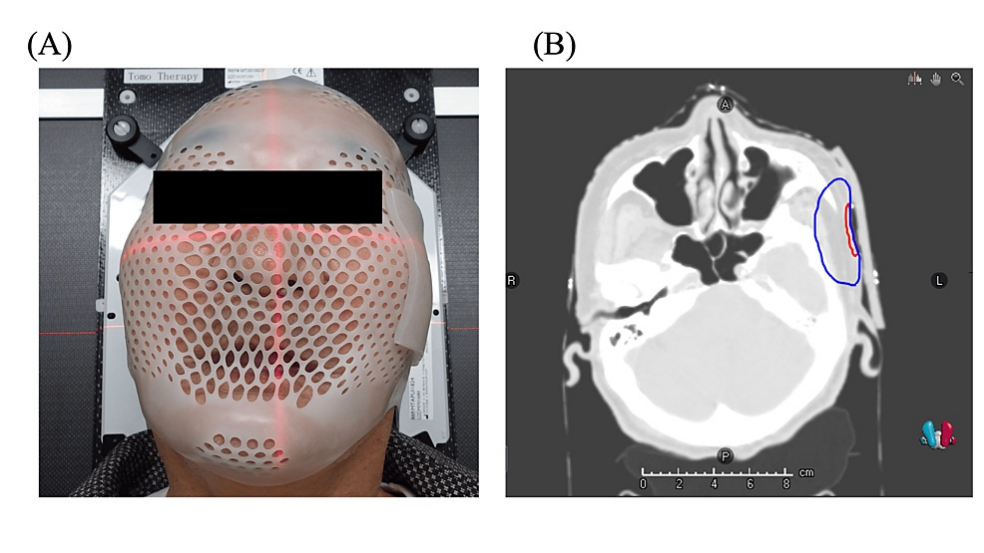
Radiotherapy setup using the HM bolus. (A) The HM bolus adhered closely to the facial irregularities. (B) Axial computed tomography image. The red line indicates the tumor bed and the blue line indicates the planning target volume. The airgap, the distance between the skin and the HM bolus, was 2.7 mm.
